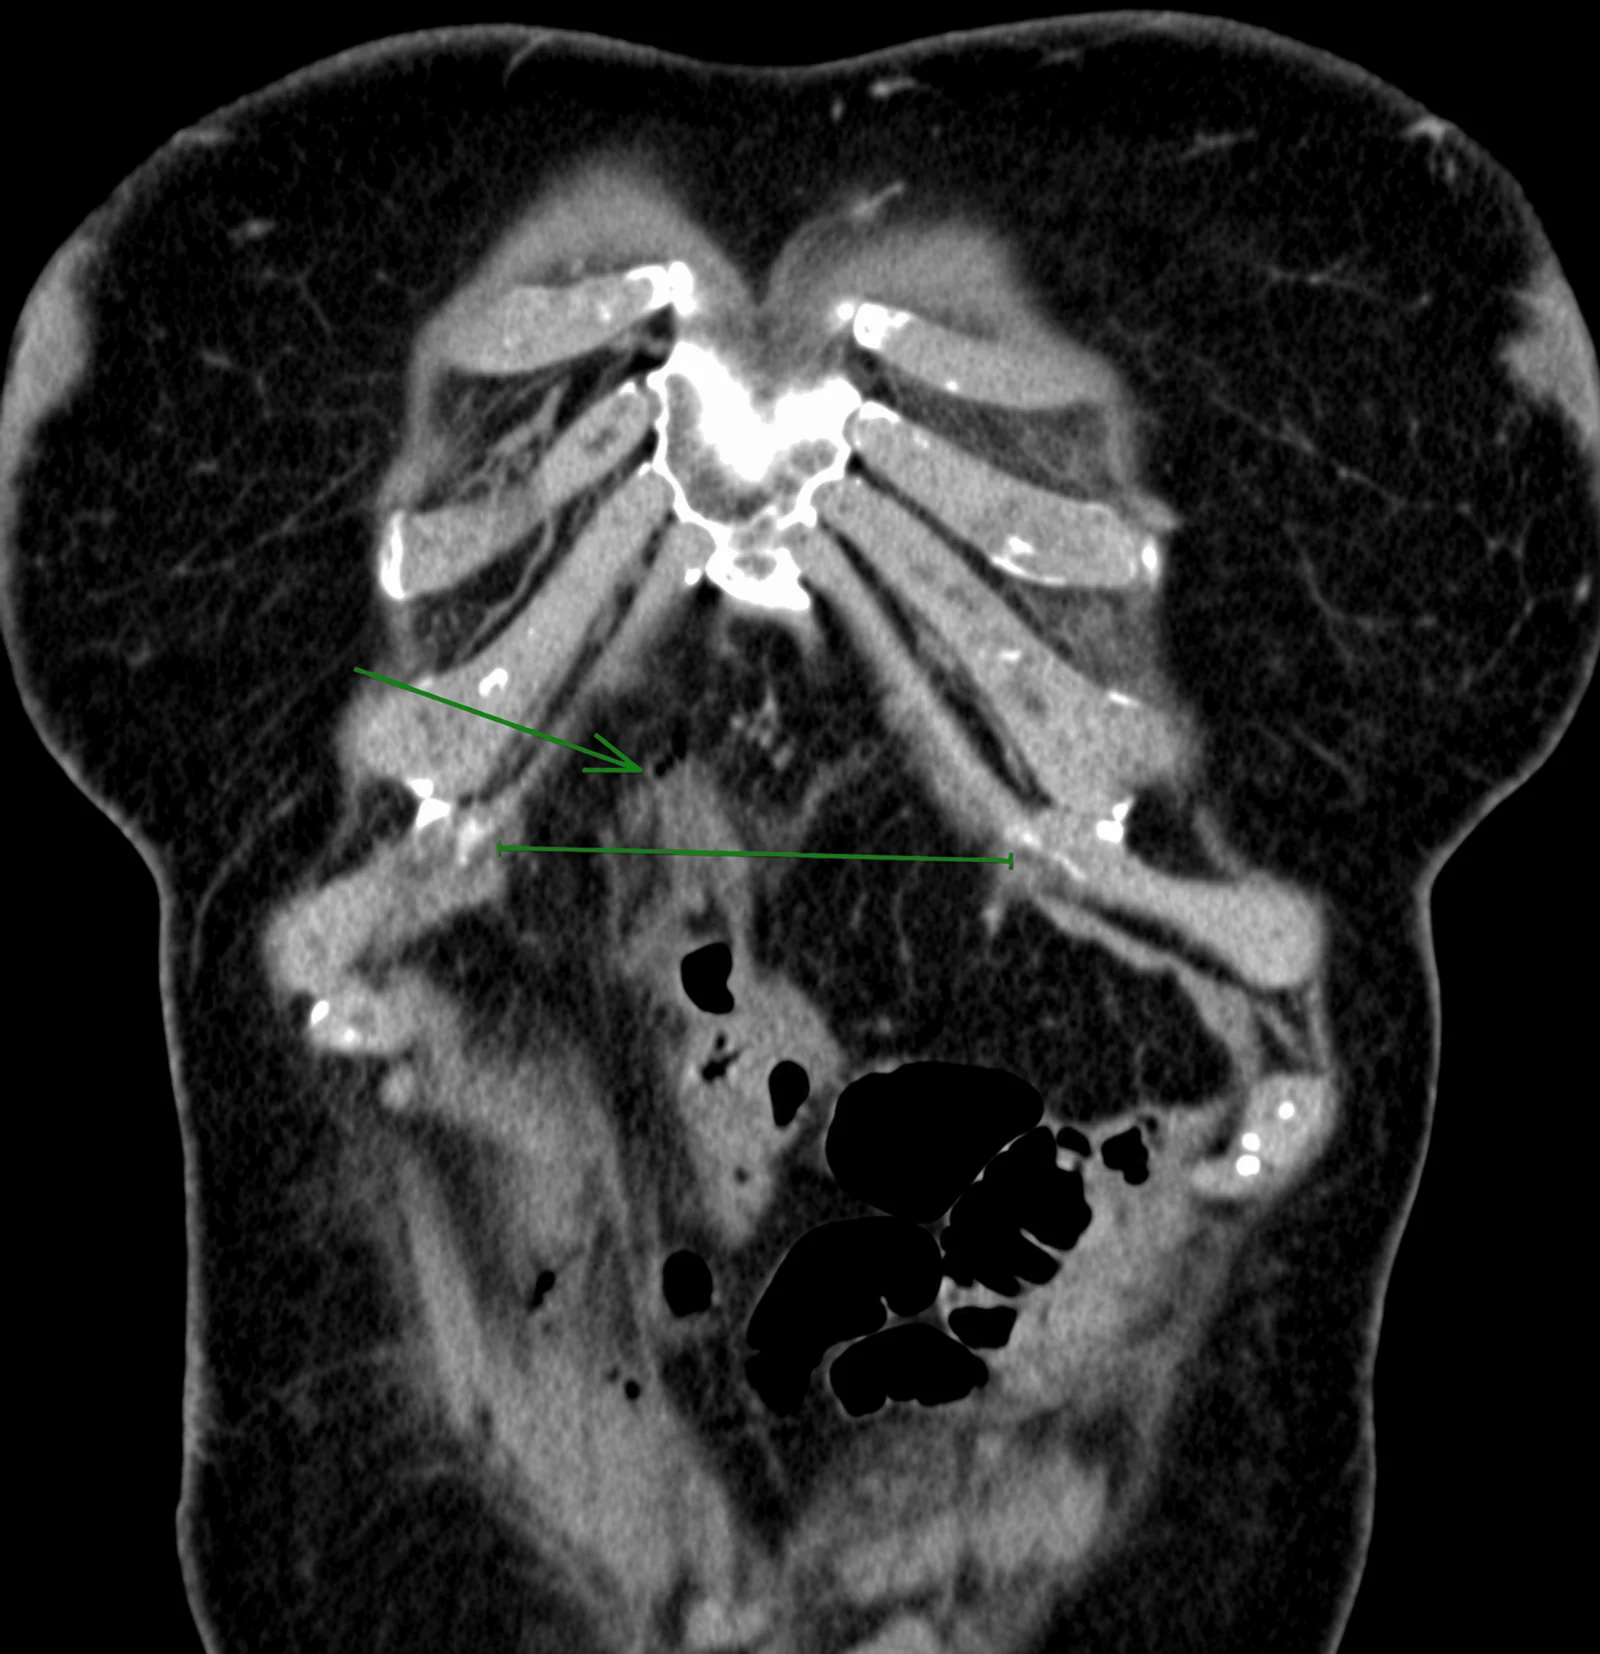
CT of the chest and abdomen. Anterior paramedian retrosternal diaphragmatic defect measuring up to 91 mm, with herniation of adipose tissue and transverse colon.
